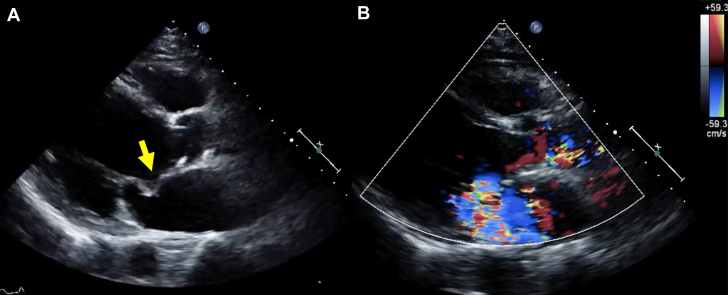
TTE and Color Doppler Image TTE (A) and color Doppler image (B) showing thickening of the anterior mitral leaflet (yellow arrow) and severe mitral regurgitation. TTE = transthoracic echocardiogram.
